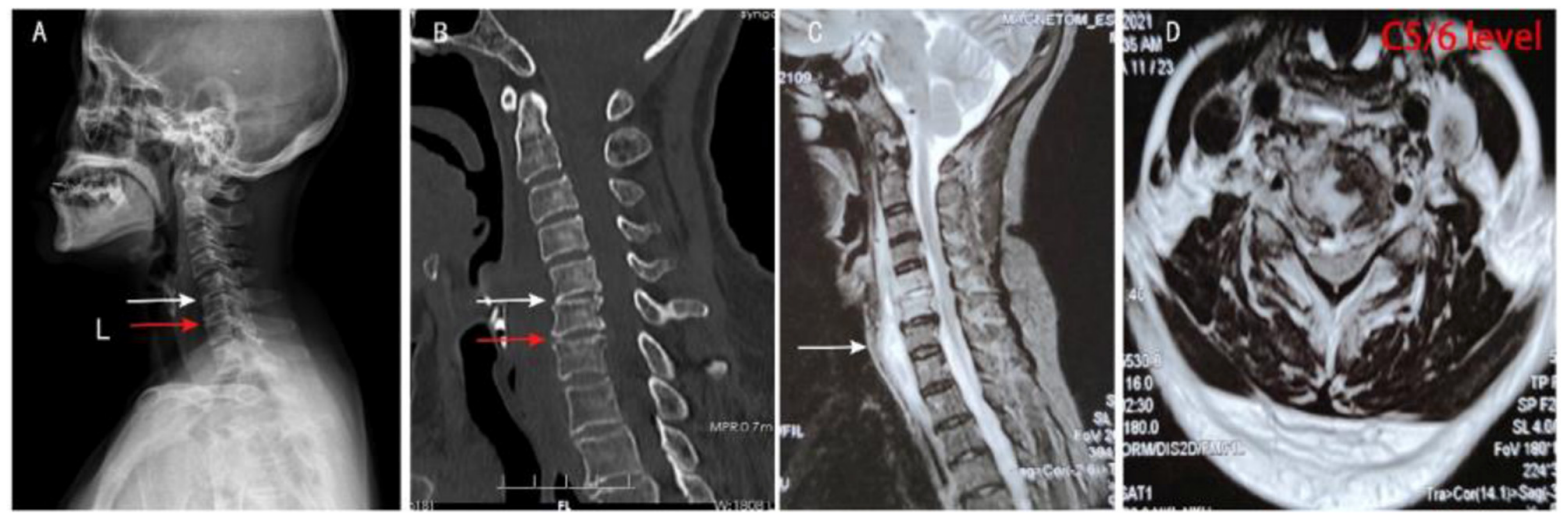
Preoperative imaging results of the patient. (A,B) Preoperative cervical spine X-ray and CT showed narrowing of the C5/6 (white arrow) and C6/7 (red arrow) intervertebral space, poor bone structure at the upper and posterior edges of the C6 vertebral body, and thickened soft tissue at the anterior edge of the C4-T2 vertebral body. (C,D) Preoperative sagittal and transverse MRI of the cervical spine showed the abscess was located in front of the C2-T2 vertebral body (white arrow) and also in the C5/6 intervertebral space, both C5 and C6 vertebral bodies showed hypersignal changes.
